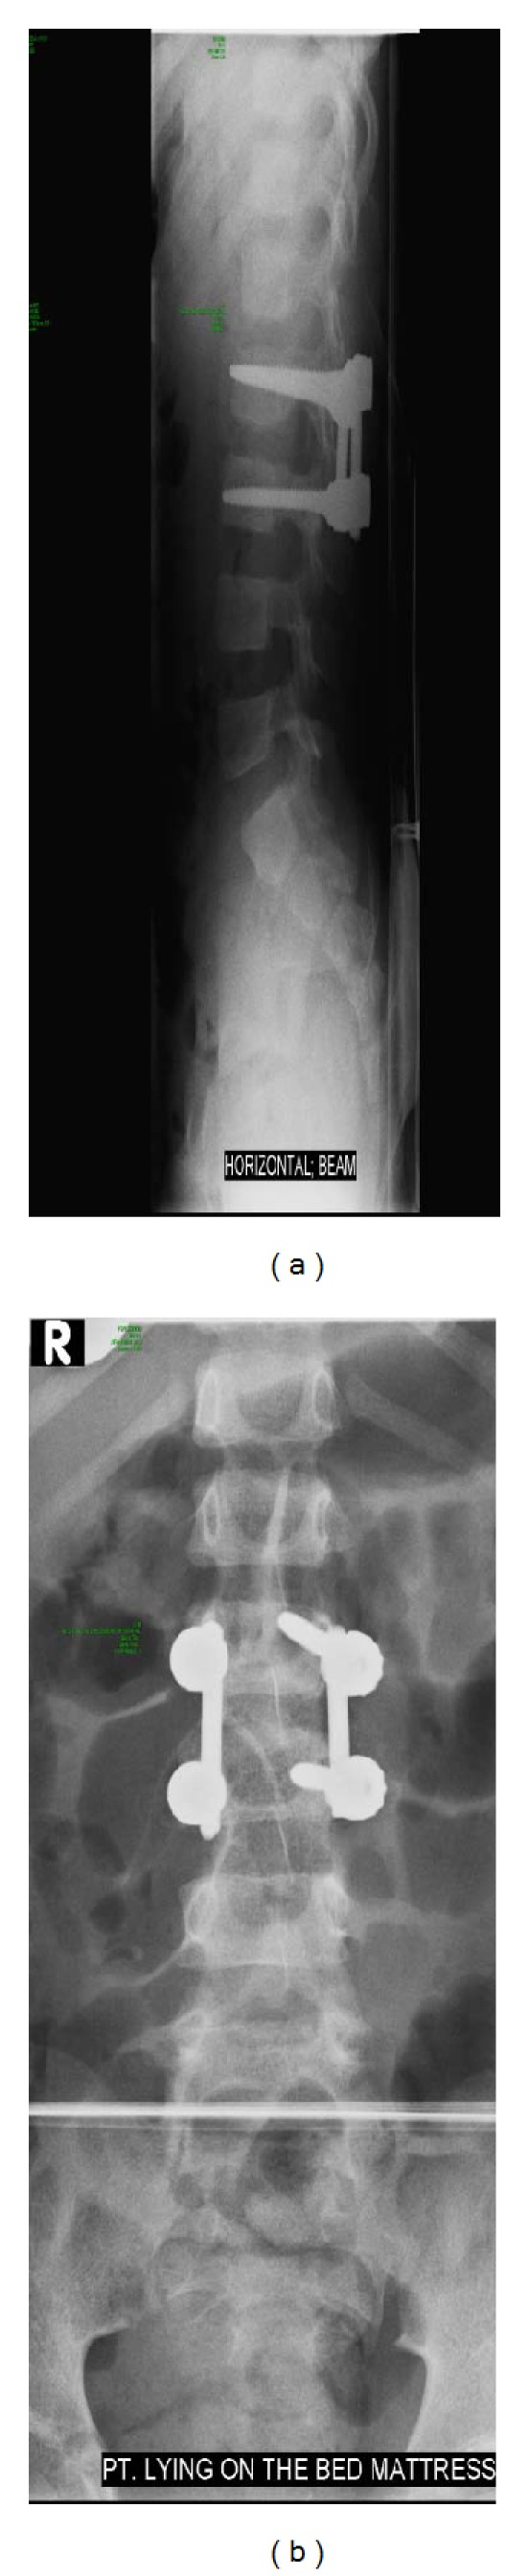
Lateral (a) and anteroposterior (b) radiographs of the lumbar spine after decompression of the spinal cord and fusion of second and third vertebral bodies (L2-L3).
